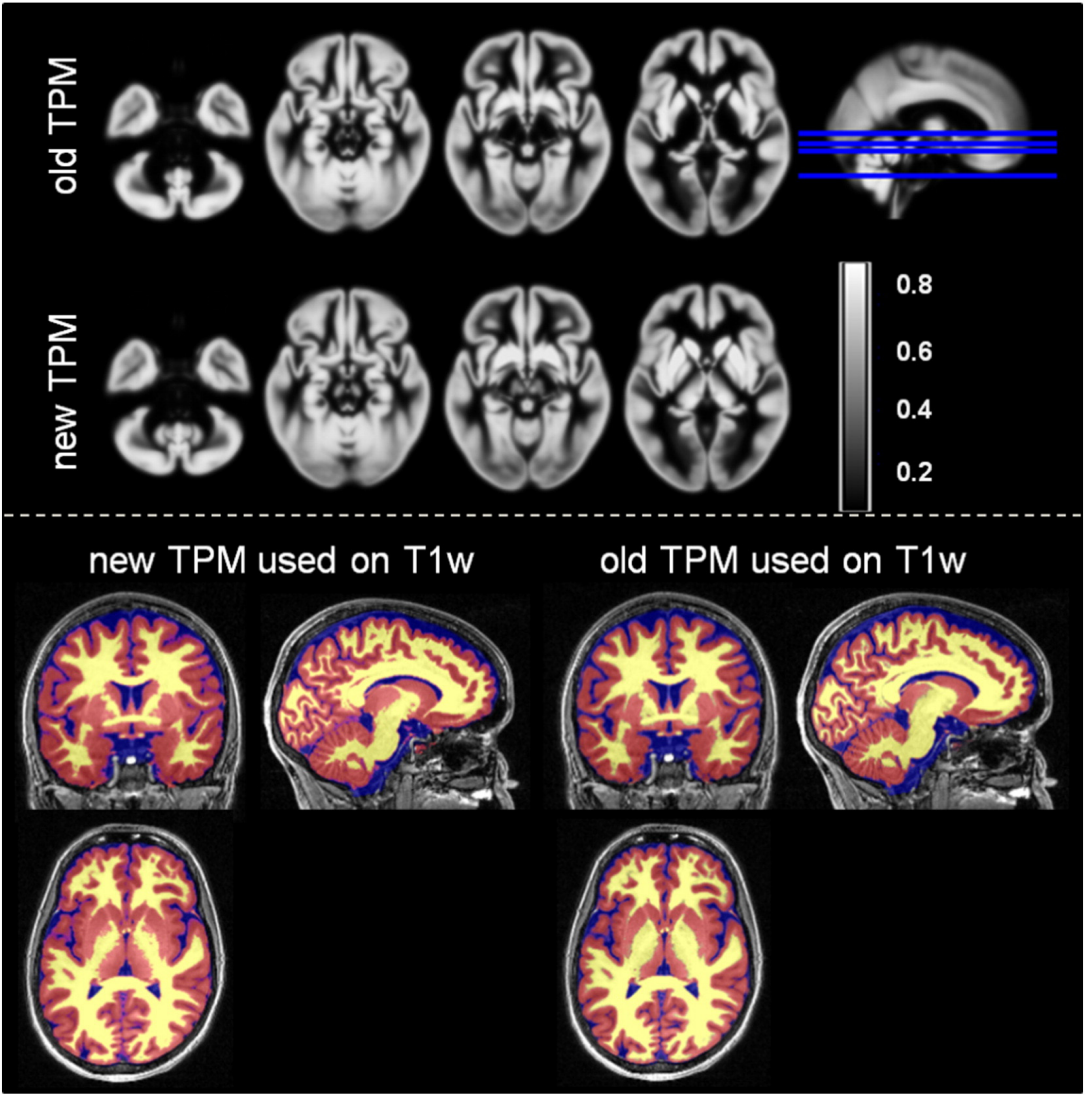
Top panel: axial view on the new and the conventional tissue probability maps (TPMs) for gray matter. Bottom panel: example of tissue classification from T1-weighted data using the new and the conventional TPMs. The gray matter probability maps is represented in red, the white matter—in yellow and the cerebro-spinal fluid (CSF)—in blue.
